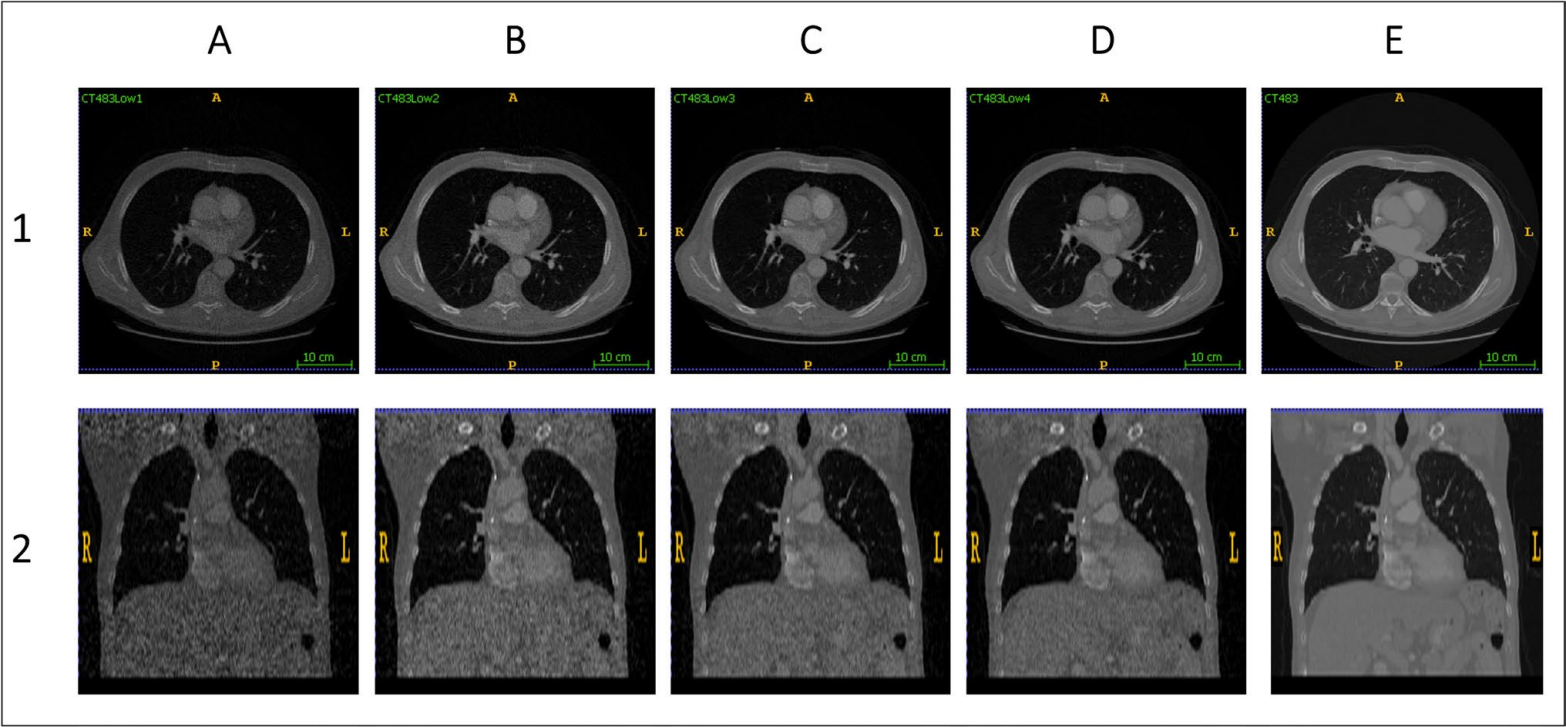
Transaxial and coronal views of A 10% low-dose, B 20% low-dose, C 40% low-dose, D 60% low-dose, and E full-dose (100%) CT images in the first and second rows, respectively
